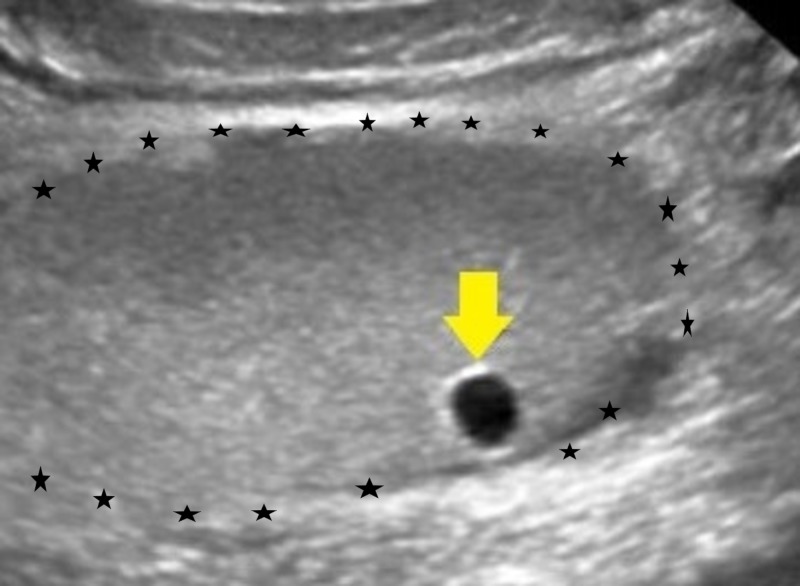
The ultrasound image showing the renal cyst (yellow arrow) in the right kidney (star border)
